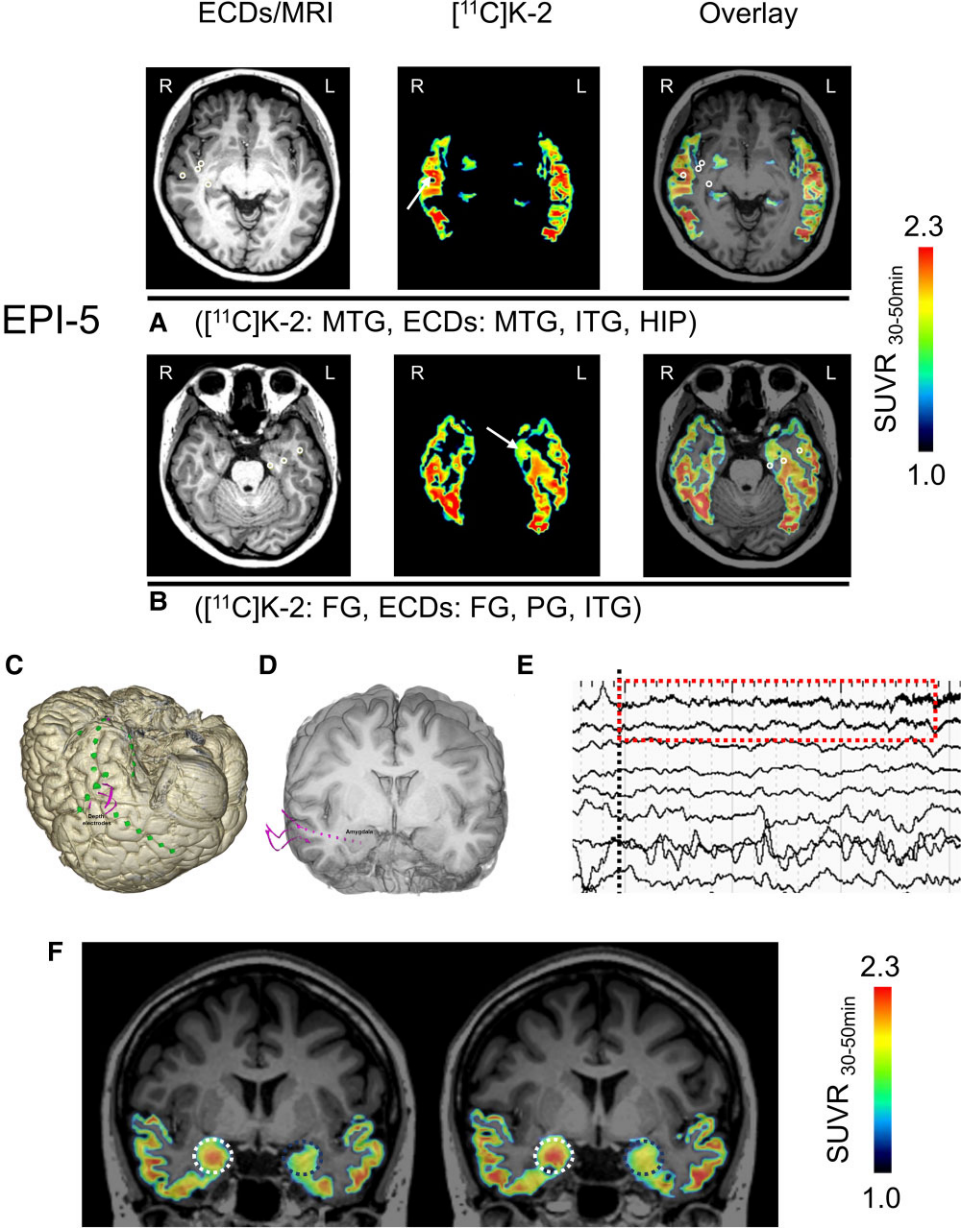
[^11^C]K-2 is highly taken up in the SOZ. (A and B) Representative [^11^C]K-2 SUVR_30–50 min_ images are shown, restricted to temporal lobes where ECDs are estimated. Each panel shows ECDs on MRI (left), [^11^C]K-2 (middle) and overlay (right). White arrows indicate the area showing elevated [^11^C]K-2 uptakes and white circles indicate estimated ECDs. EPI-5 showed elevated [^11^C]K-2 uptake and estimated ECDs in the right MTG and, further, other ECDs were estimated in right ITG and HIP (A). Furthermore, elevated [^11^C]K-2 uptake and estimated ECDs were identified in the left FG, and other ECDs were estimated in left PG and ITG (B). (C) The area where subdural electrodes are implanted in EPI-5. Pink lines show depth electrodes and green dots show brain surface electrodes. (D) Three depth electrodes were implanted targeting to right amygdala (pink lines and dots). (E) The iEEG change at the seizure onset (ictal EEG onset) is shown with black dots line. The earliest iEEG change (low-voltage fast activity indicated by red-dashed square) was detected by the depth electrode in right amygdala alone. The activity was the first unequivocal change at the ictal onset phase. Therefore, we deemed that the amygdala was the SOZ in this patient. (F) The zoomed image of red-dashed square in Fig. 2E shows that low-voltage fast activity occurs in the right amygdala. (G) SUVR_30–50 min_ images show that the elevated [^11^C]K-2 uptake colocalizes with the SOZ (right amygdala: white dots circle). The contralateral amygdala is indicated as a dotted blue circle.
